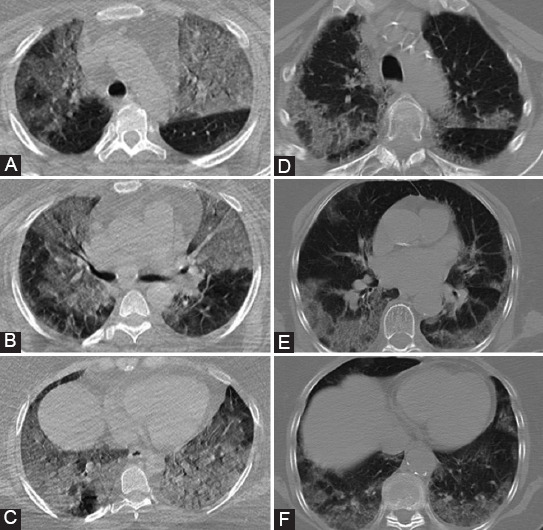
(A-C) 40-year-old woman with coronavirus disease 2019 (COVID-19) pneumonia. Non-contrast computed tomography (CT) was performed on day of admission. Chest CT images showed large areas of bilateral consolidation and ground-glass opacities in bilateral lungs and air bronchogram. The lesions involved in five lung lobes and the Zonal CT Visual Score was 44. Patient was admitted to the intensive care unit (ICU). (D-F) Axial CT images of a 72-year-old woman with COVID-19 pneumonia revealed bilateral consolidation, ground-glass opacities with mainly peripheral distribution, and accompanying reversed halo sign. The lesions involved in five lung lobes and the Zonal CT Visual Score was 27. Patient was admitted to the ICU.
